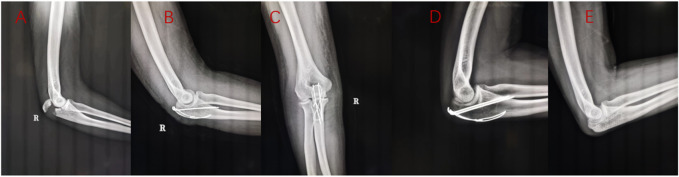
A 32-year-old female patient with right olecranon fracture caused by falling injury. Preoperative X-ray examination (A), X-ray examination 2 days after Kirschner wire tension band fixation (B, C), 3 months X-ray examination (D),1 year later, the internal fixation was removed (E).
